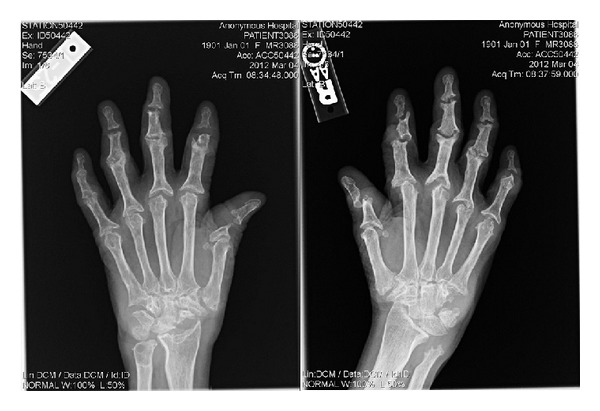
X-ray of the hand showing the “pencil-in-cup” phenomenon and narrowing of the joint spaces.
